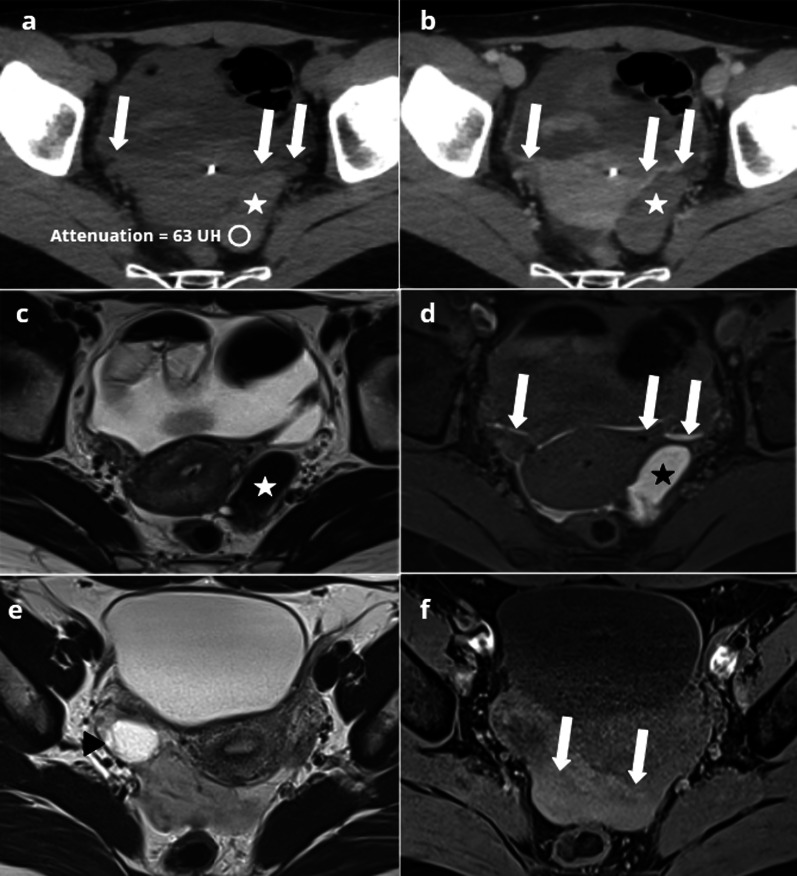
42-year-old female presenting with a non-febrile acute pelvic pain, with negative human chorionic gonadotropin (HCG) and C-reactive protein (CRP). Non-enhanced CT (a) showing a spontaneously dense left ovarian mass (star) with free intraperitoneal fluid (arrows). After contrast injection (b), no enhancement of the mass is observed. MRI in the same patient (c, d) showing a distorted left ovarian mass (star) in low signal on T2W images (c) and high signal in T1W images (d). Free intraperitoneal fluid (arrows) presents a hemorrhagic level showing the same signal as the endometrioma (d). 27-year-old female patient presenting with right corpus luteal cyst rupture. MRI (e, f) shows a heterogeneous cyst (arrowhead) on T2W images (e), and free intraperitoneal fluid (arrows) showing an intermediate signal on T1W images (f)
